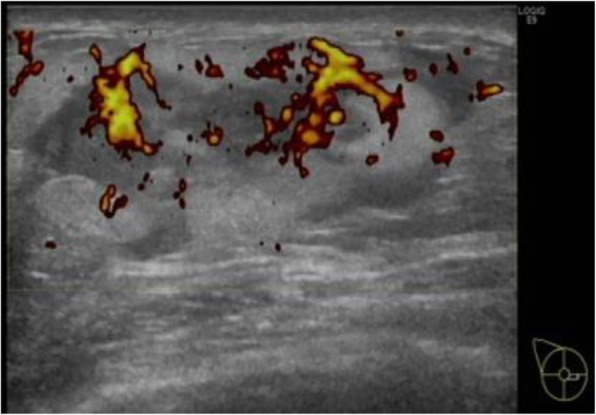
Doppler sonography showed hypervascularization of the tumor
